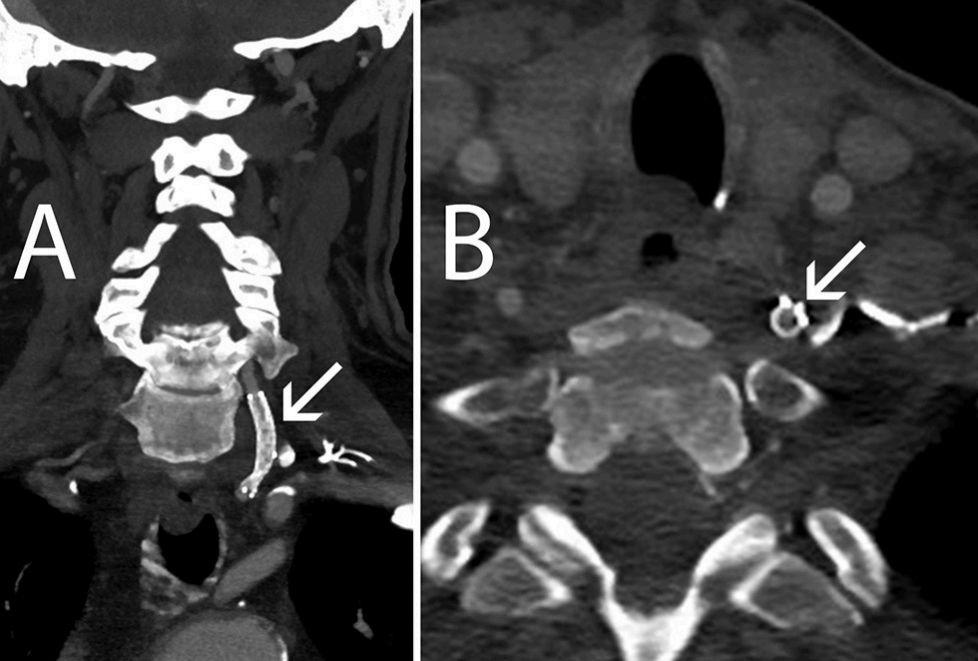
Sixteen-month follow-up CT Angiography. (A) Coronal view, left VA remains patent (arrow) (B) Axial view, the Accunet EPD (arrow) is flattened against the VA wall by the EV3 EverFlex stent.
